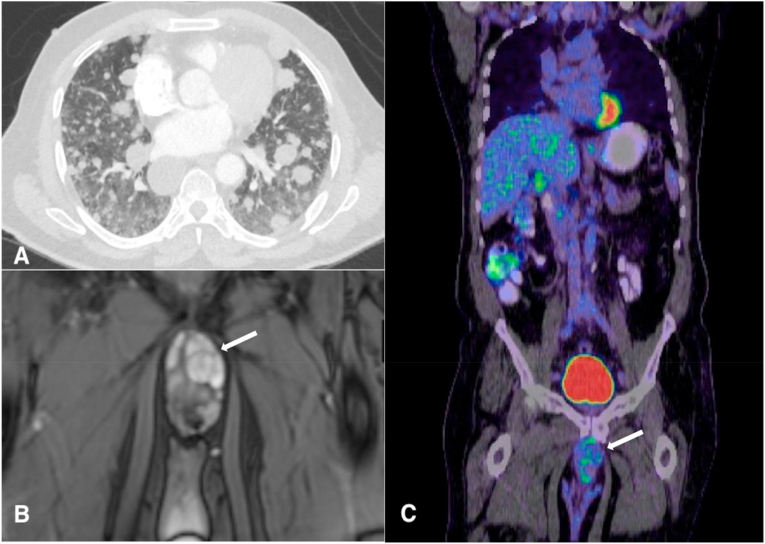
(A, B, and C): (2 A) Computerized Tomographic chest film confirming bilateral rounded pulmonary nodules. (2 B) MRI pelvis showing the penile shaft basal tumor (Highlighted by the white arrow). (2 C) Coronal PET-CT confirming the penile uptake (Highlighted by the white arrow).
